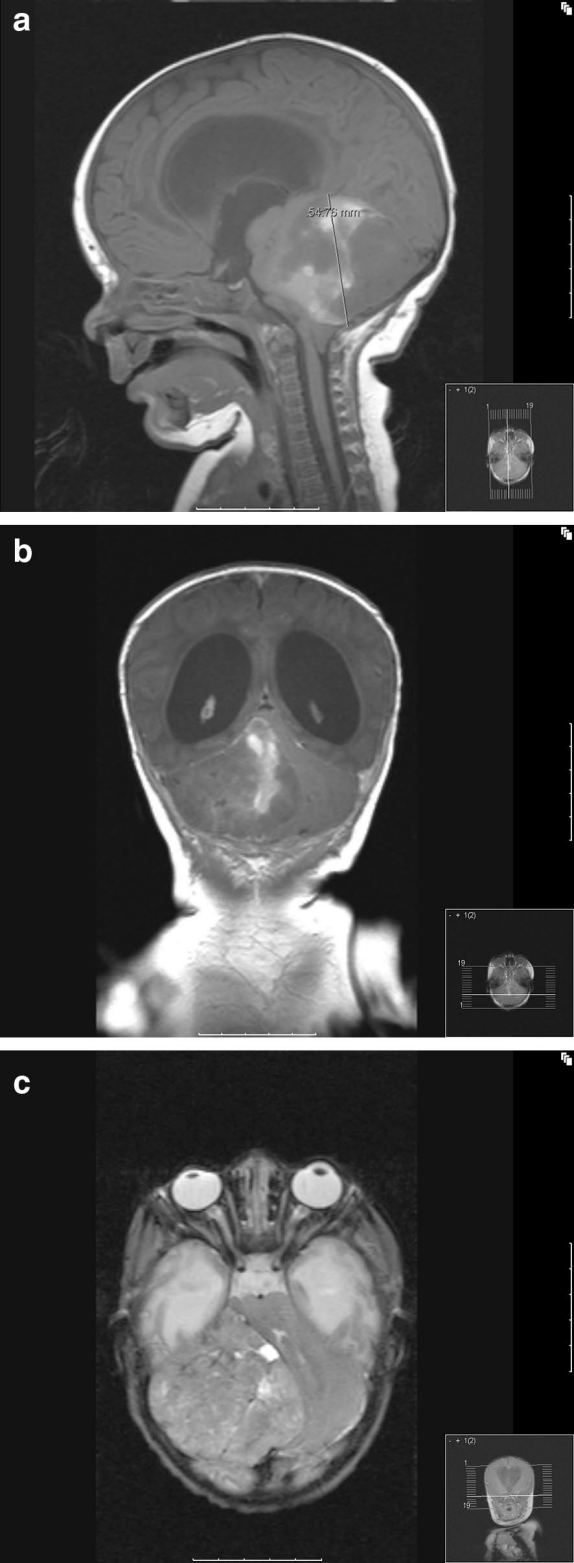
MRI scans disclose a partially contrast enhancing tumor to the right in the posterior fossa with marked displacement of the brain stem to the *left*. a sagittal, b axial and c coronal scans.
